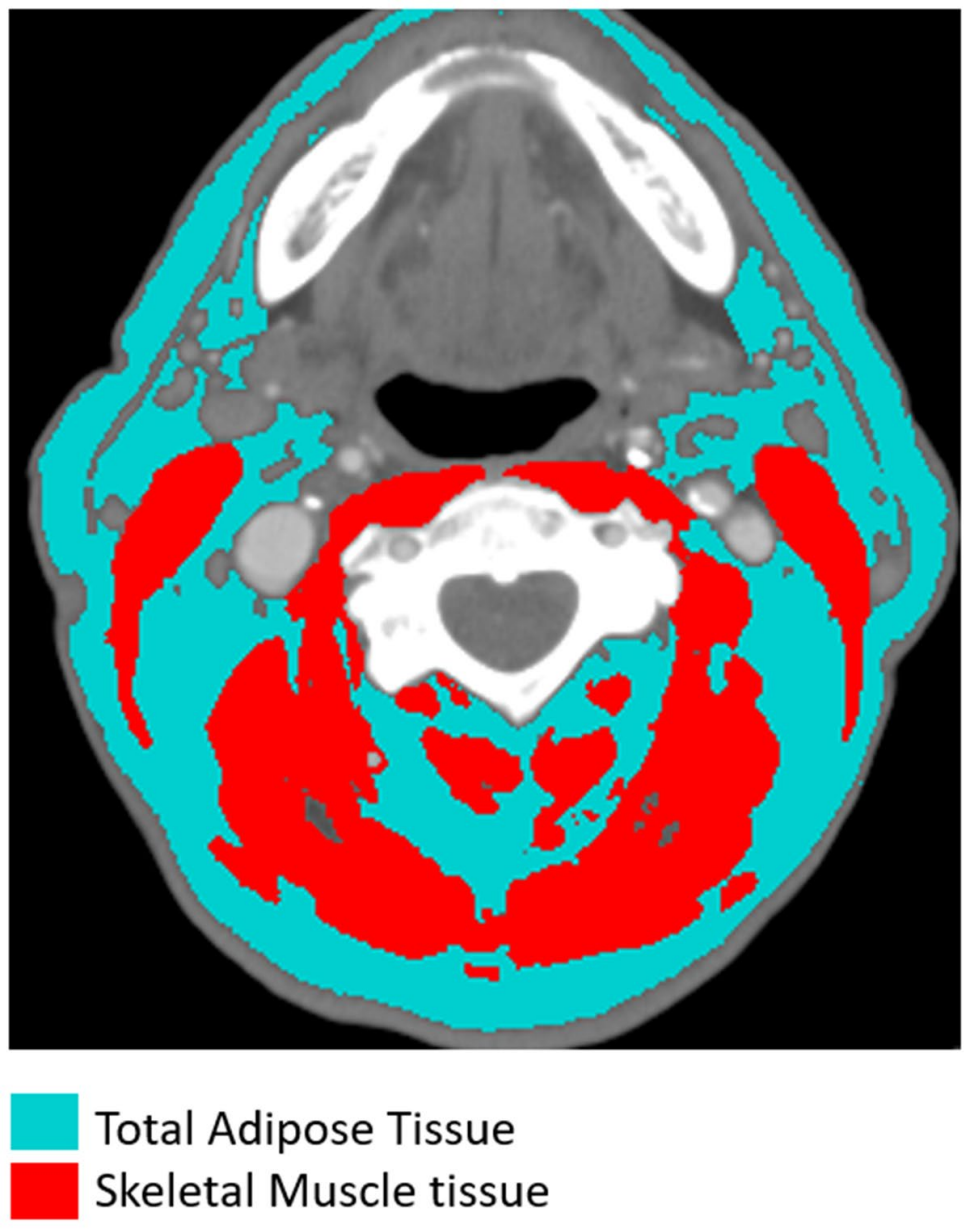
Segmentation of total adipose tissue (with subcutaneous and internal tissues highlighted in blue) and skeletal muscle tissue (including the sternocleidomastoid and paravertebral muscles highlighted in red) at the level of the third cervical vertebra (C3) in CT images.
